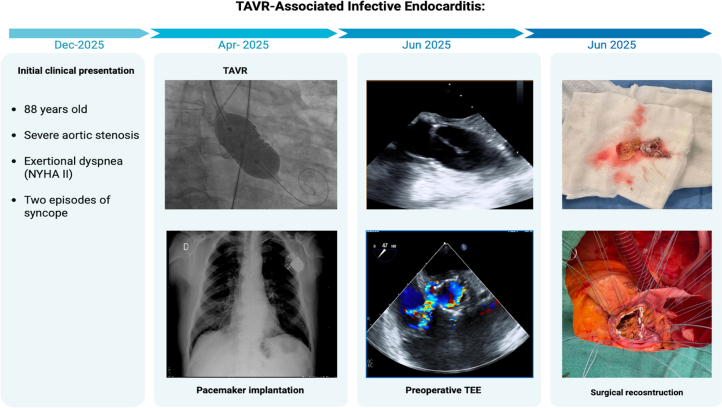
Timeline of Case Presentation TEE = transesophageal echocardiography; TAVR = transcatheter aortic valve replacement.
